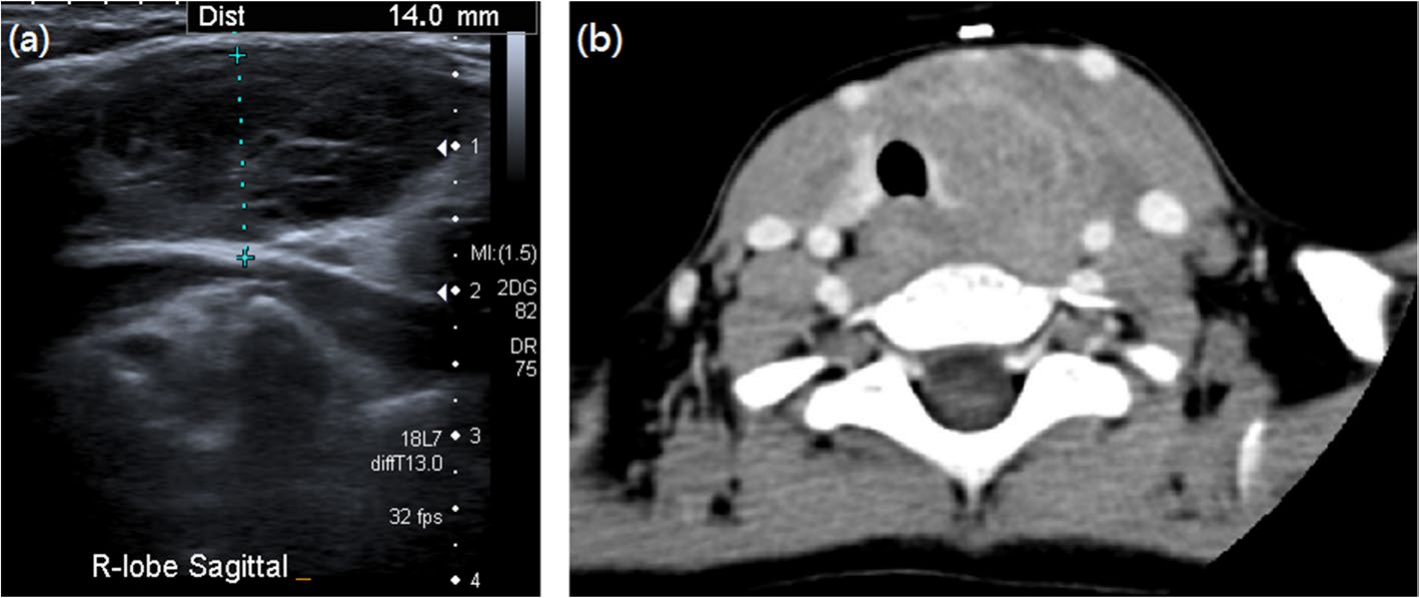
a Thyroid sonography shows multiple ill-defined heterogeneous hypodense nodules. b Axial contrast enhanced computed tomography of thyroid Langerhans cell histiocytosis shows enlarged thyroid with ill-defined and heterogeneous hypodense lesions without enhancement in bilateral lobes of thyroid
